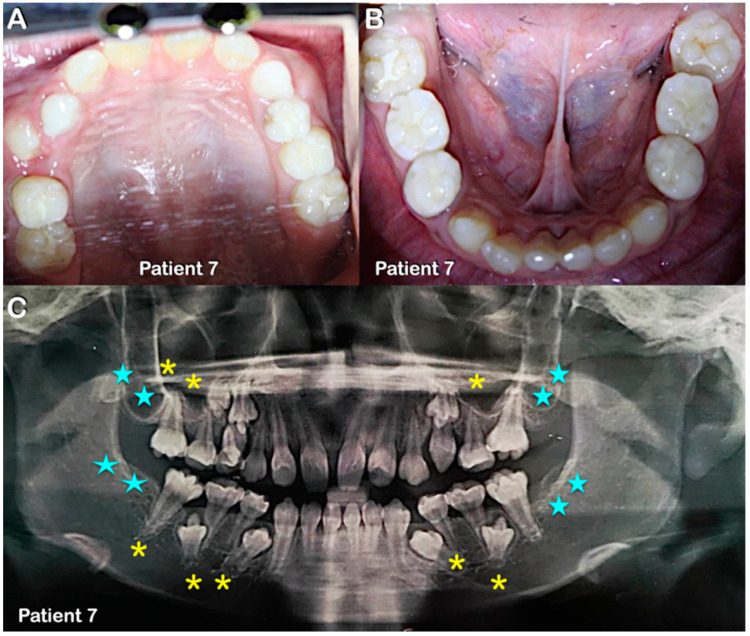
Patient 7 in mixed dentition. (A,B) Round-shaped permanent molars and molars with multiple supernumerary cusps. (C) Panoramic radiograph showing agenesis of second and third permanent molars (blue stars) and single-rooted primary and permanent molars (yellow asterisks).
